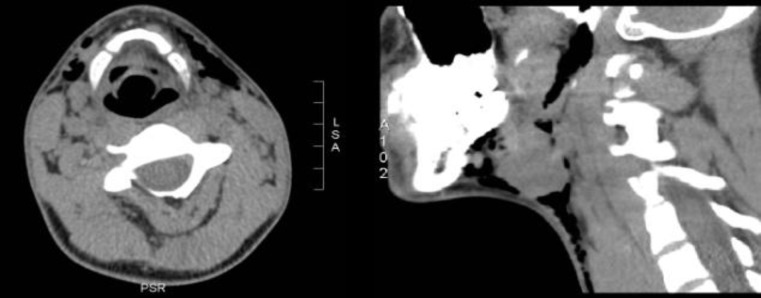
Computed tomography scan in the axial and sagittal planes showing air in the cervical subcutaneous tissues on both sides of the neck, especially on the left. There is also interruption of the air column in the left tonsillar lodge
